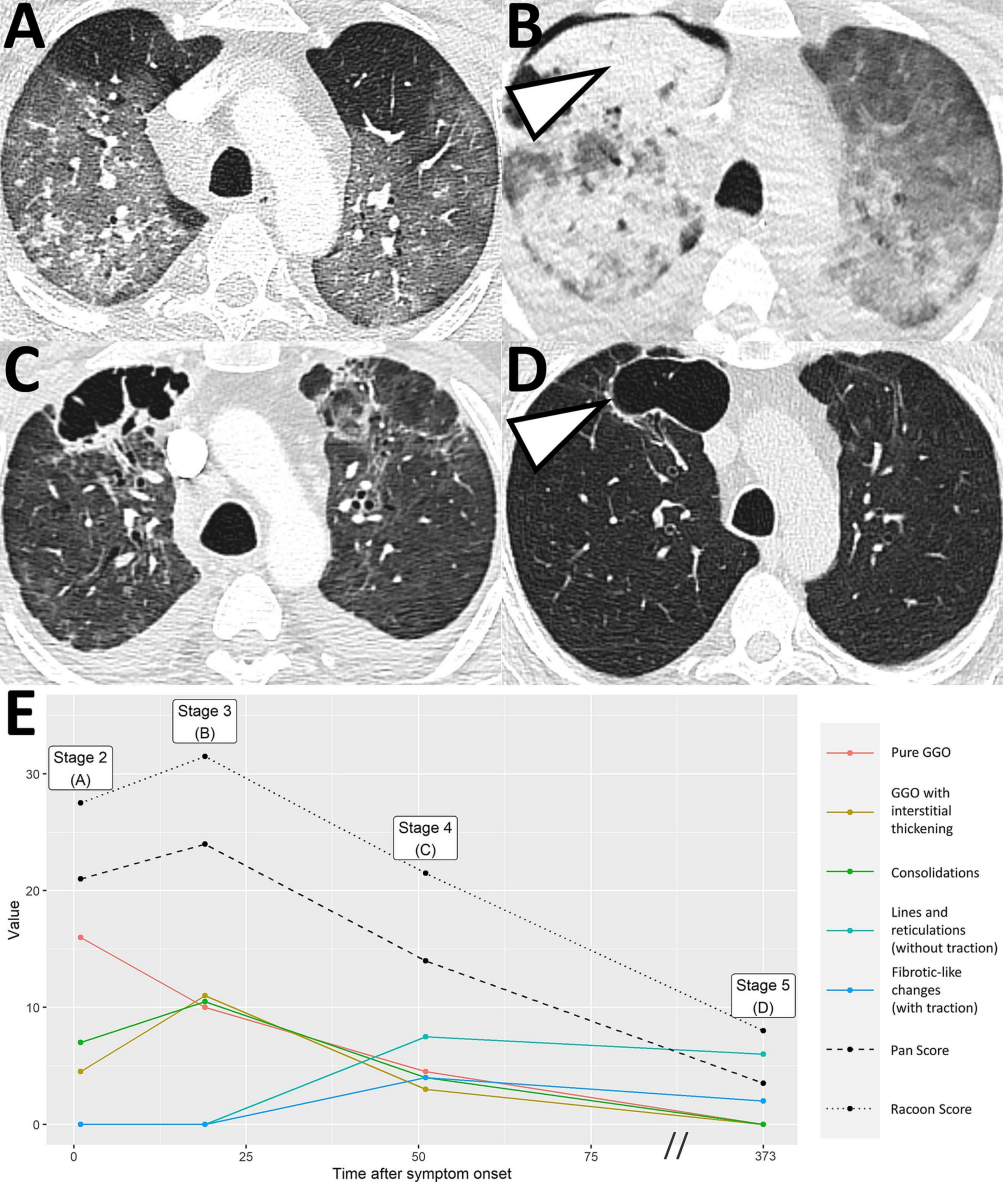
Severe COVID-19 pneumonia with post-COVID-19 sequelae. A 43-year-old male patient presented at the hospital with severe dyspnea 1 day after anamnestic symptom onset of laboratory-proven COVID-19 pneumonia. Initial chest CT demonstrated progressive stage COVID-19 pneumonia with predominantly ground glass opacities and interstitial thickening [A, RVPS = 28 (16/5/7/0/0); stage 2]. The second CT scan 19 days after symptom onset showed progression to peak stage findings [B, RVPS = 32 (10/11/11/0/0); stage 3]. Extensive consolidation indicated bacterial superinfection, which obligated for intravenous antibiotics. At the location of the dense consolidation in the right upper lobe (white arrowhead in B), a third CT scan 51 days after symptom onset reported the formation of a cystic, presumably irreversible lung tissue damage. Yet, there were still findings consistent with infectious pneumonia [C, RVPS = 22 (5/3/4/8/4); stage 4]. Follow-up imaging in the post-infectious interval at day 373 after symptom onset confirmed the assumption of an irreversible parenchymal defect [white arrowhead in D, RVPS = 8 (0/0/0/6/2); stage 5]. The Pan score and RVPS demonstrate a parallel course (E). However, the RVPS recognizes the formation of post-COVID-19 CT findings (blue line in E), which are not appreciated by the Pan Score. RVPS, RACOON Viral Pneumonia Score; GGO, ground glass opacities.
